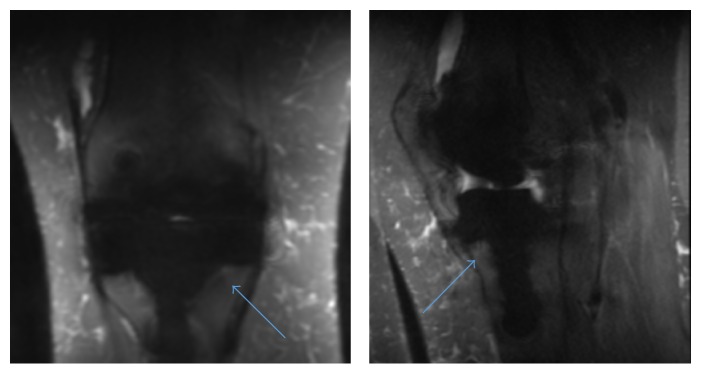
Coronal and Sagittal MAVRIC® STIR SLR MRI showing complete resection and resolution of BMLs 12 months after TKR surgery (blue arrows).
